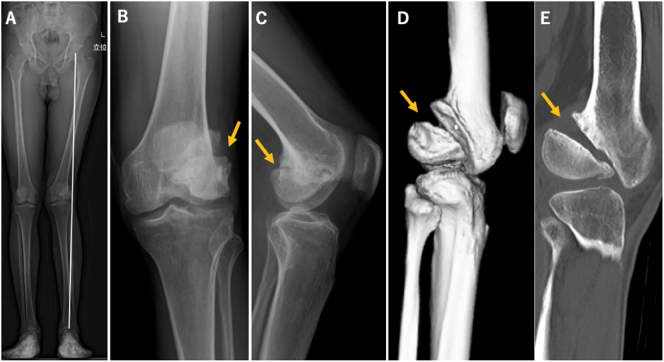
Conventional X-ray and computed tomography showed nonunion of the lateral Hoffa fracture. (A) Whole standing lower limb radiograph showed valgus deformity in standing position. (B) Anteroposterior view. (C) Lateral view. (D) 3D computed tomography (CT) reconstruction. (E) CT sagittal view.
